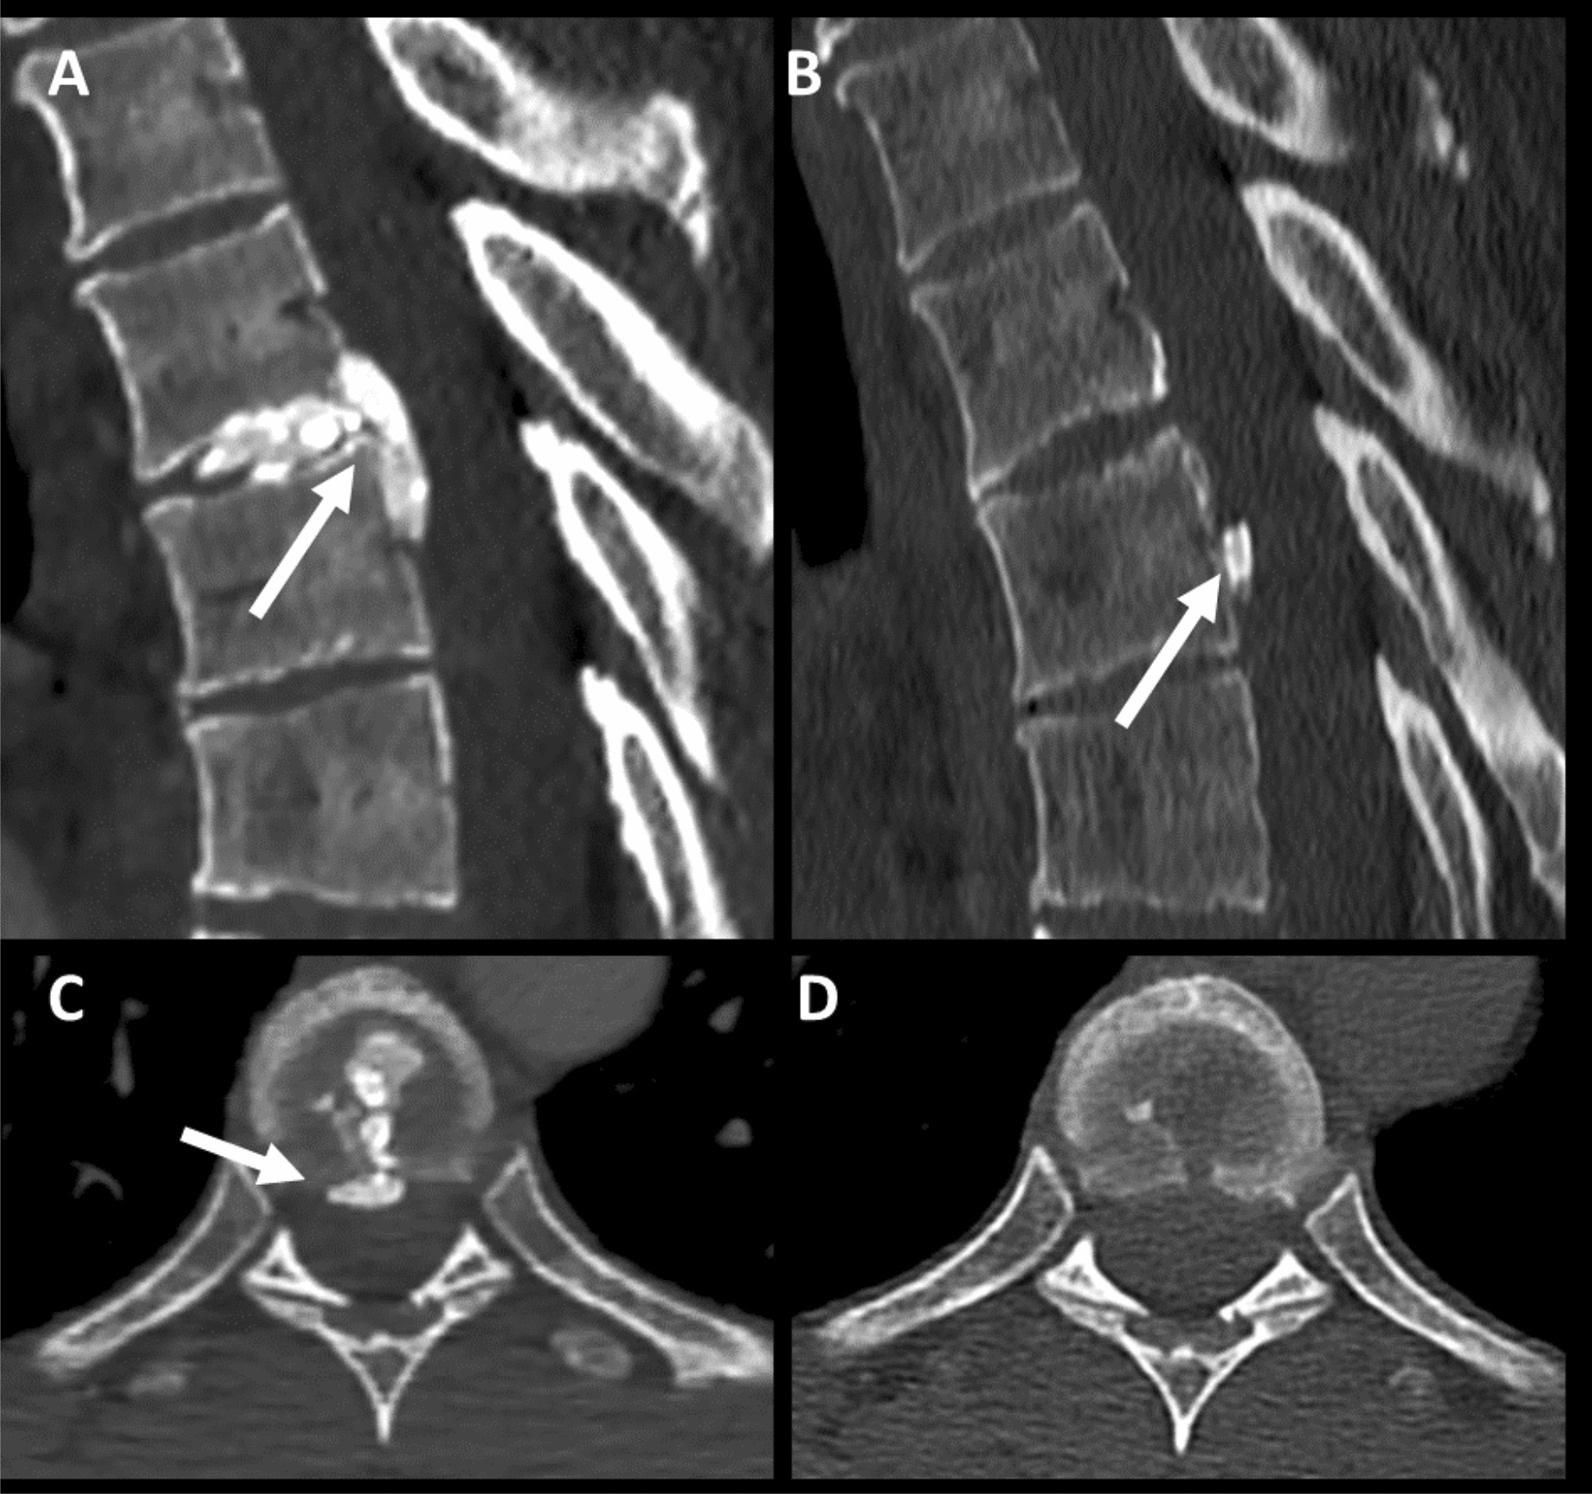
Findings on CT scan. Calcified posteromedial thoracic disc extrusion slightly lateralized to the left at the T5-T6 level with an 18 mm craniocaudal migration (arrows), abutting the anterior aspect of the spinal cord, without significant canal stenosis (A, C). Six months later, spontaneous decrease of the disc extrusion with a residual calcification adjacent to the posterior wall of T6 (B, D)
